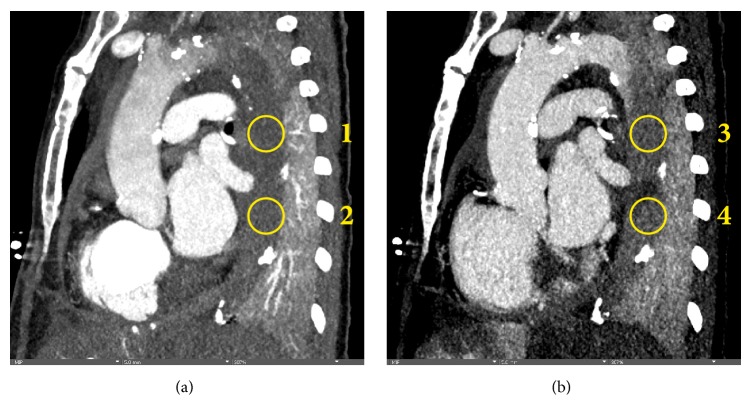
Acute aortic occlusion. Computed tomography. (a) Sagittal plane, arterial phase after intravenous injection of contrast agent. The descending aorta is not filled with contrast (circle 1: 70 HU, circle 2: 73 HU). Note the severe calcification of the aorta. (b) Sagittal plane, late phase after intravenous injection of contrast. While few contrast enters the proximal part of the thrombus (apposition zone, circle 3: 105 HU), it does not reach the already more solid parts of the thrombus (circle 4: 78 HU). HU: Hounsfield Units.
